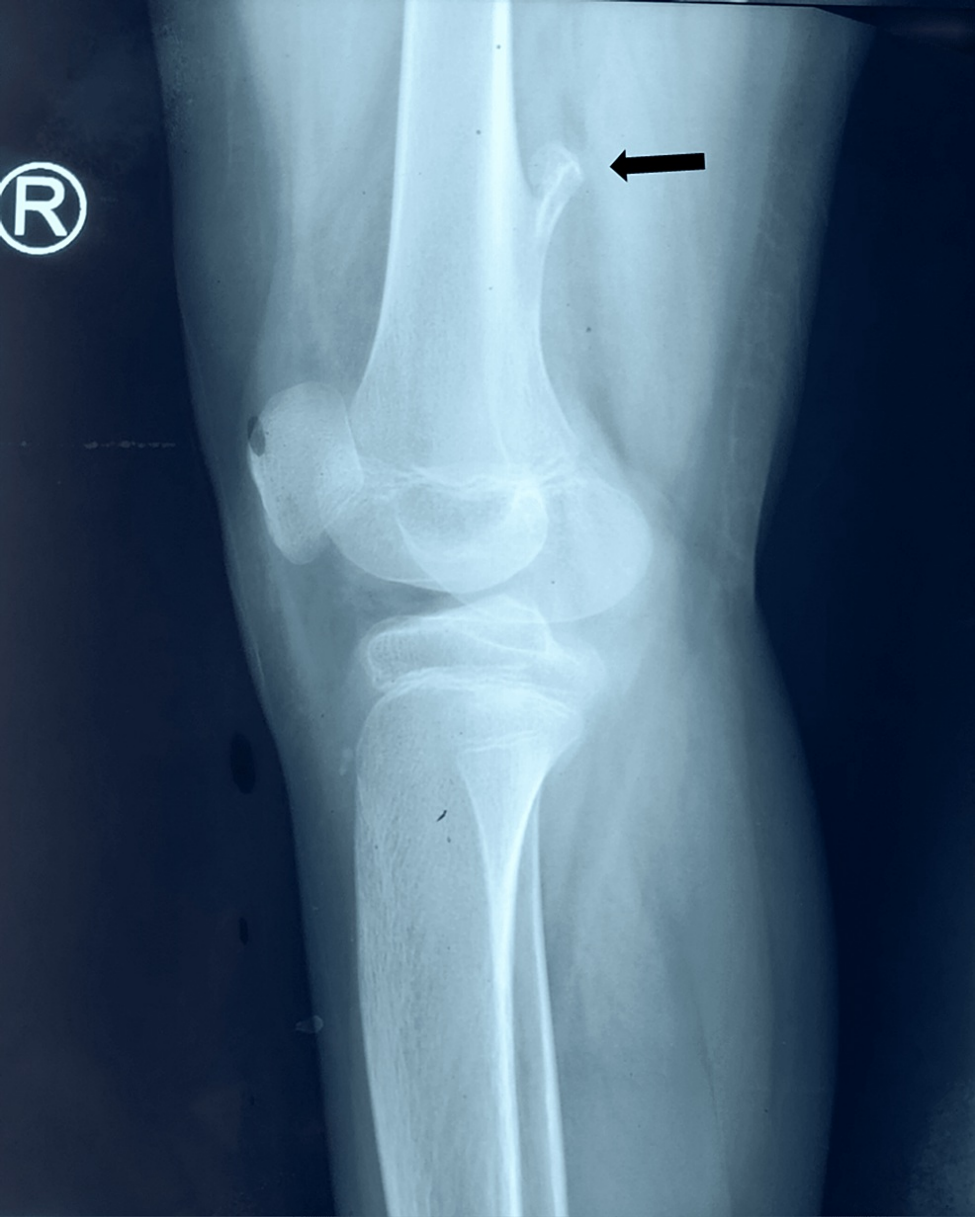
X-ray of the right knee (lateral view) showing bony exostosis in the right femur (black arrow)
